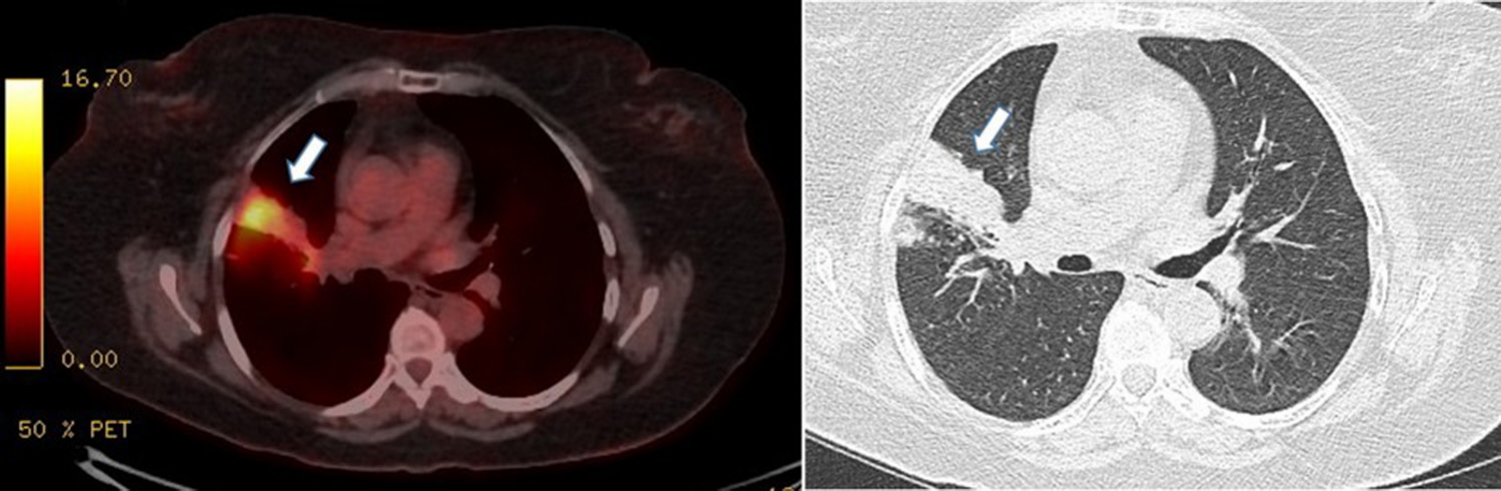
Computed tomography scans of a healthcare workers with occupationally transmitted extensively drug-resistant tuberculosis, France. Scan revealed hypermetabolism (standardized update value 9.1) of the right upper lobe alveolar consolidation, indicated by white arrows.
